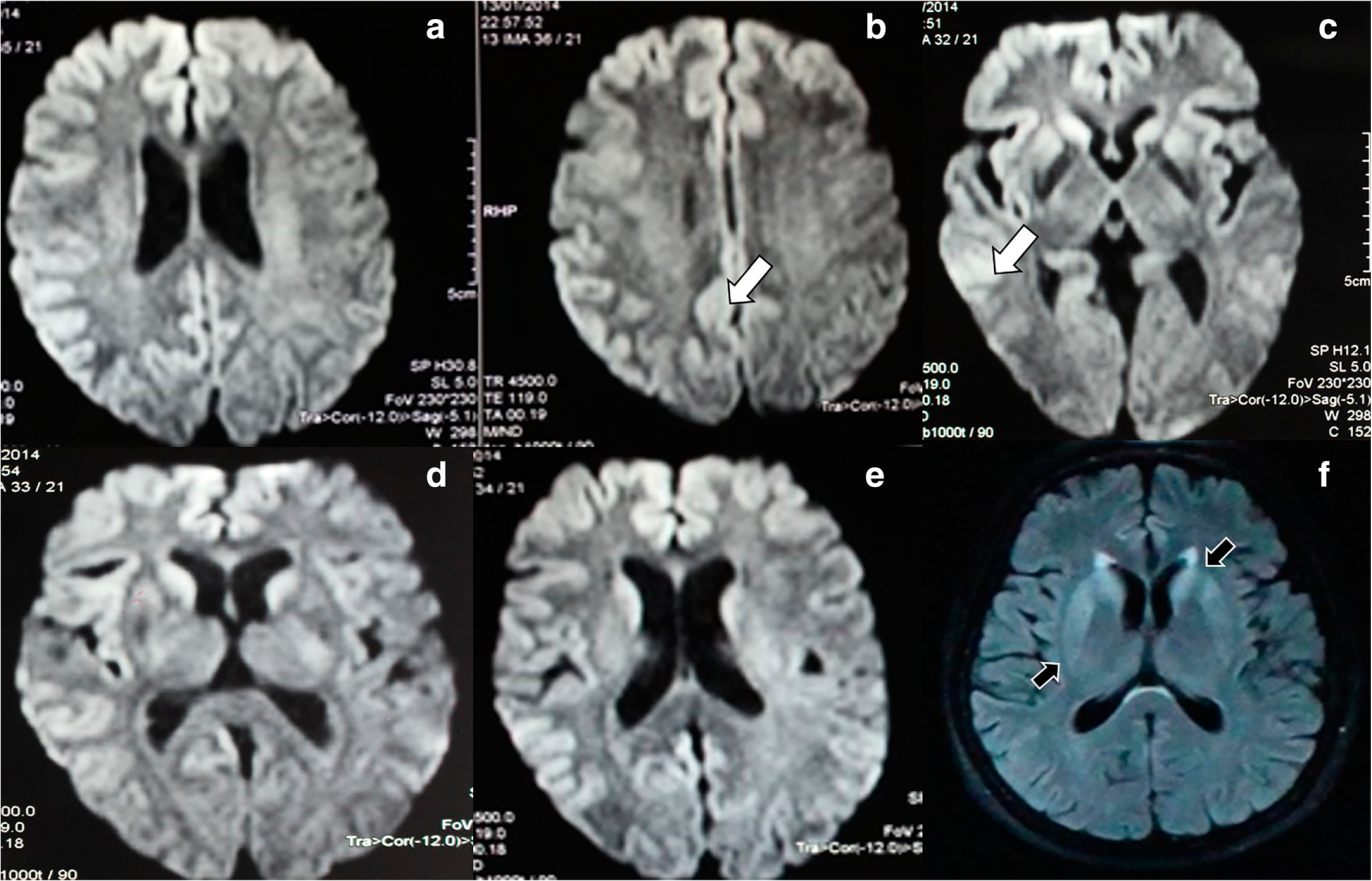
Imaging of the patient in case 2 3 months after onset of sporadic Jakob-Creutzfeldt disease. a-e, Axial diffusion-weighted imaging (DWI). Bilateral restricted diffusion cortical ribboning is shown in the bilateral temporal and parietal cortices (white arrows). f, fluid attenuated inversion recovery (FLAIR) shows high signal abnormalities in caudate and putamen nucleus (black arrows)
